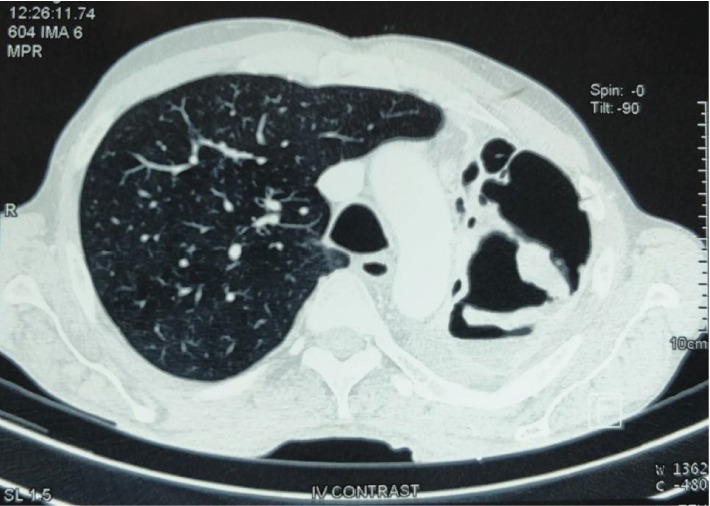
CT scan (lung window) showing a completely destroyed left lung with multiple air- and fluid-filled cavities and fibrotic bands.
